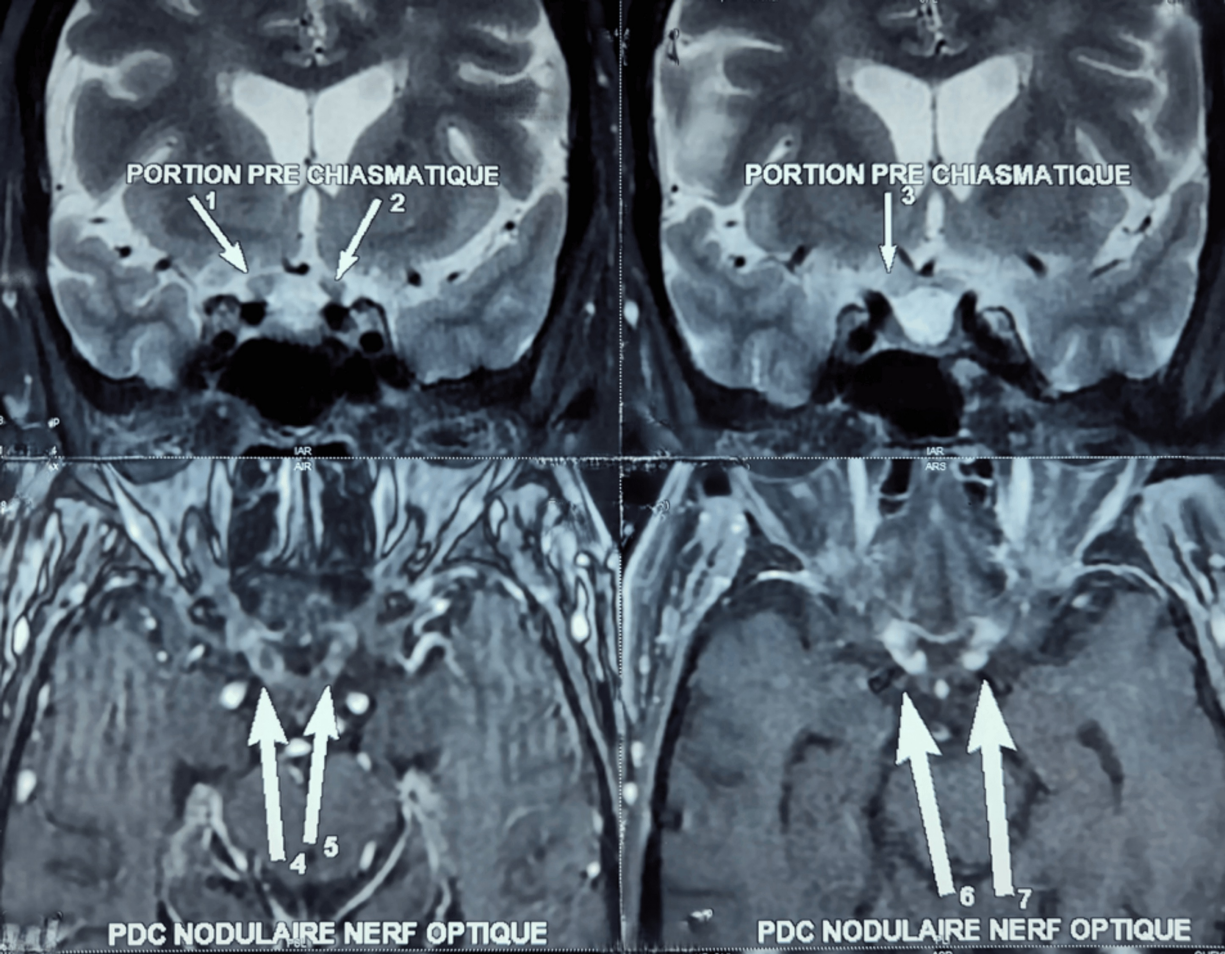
MRI images showing optic nerve thickening
